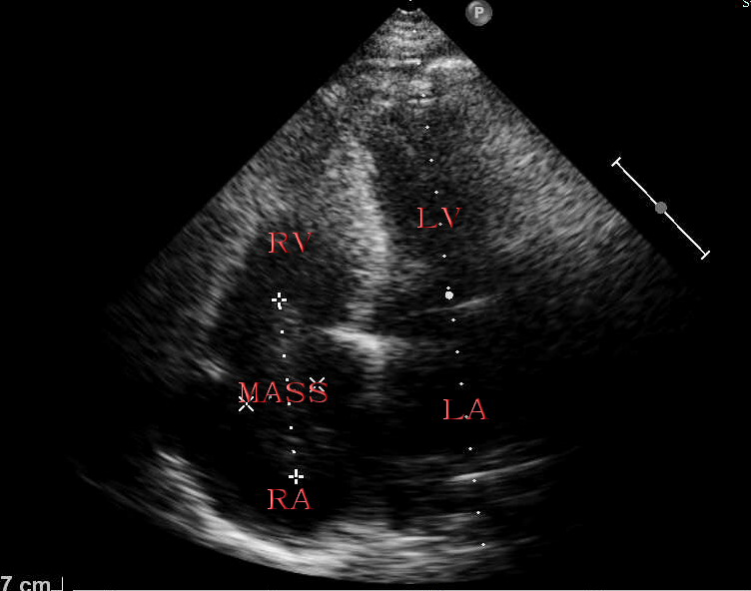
Apical four chamber view showing right atrium filled with a medium echogenic oval tumor mass by transthoracic two-dimensional echocardiography. RV: right ventricle, RA: right atrium, LV: left ventricle, LA: left atrium.
